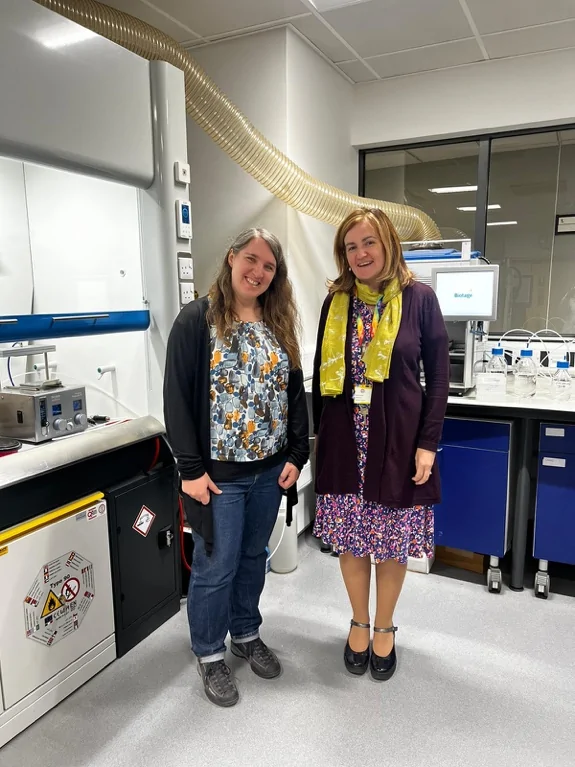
CTLS Job Shadowing Programme, Visit Testimonial 2. Dr. Maria Doppler (left) and Dr. Natalie Homer (right) during Maria’s visit to the Mass Spec Core Facility at the University of Edinburgh.
